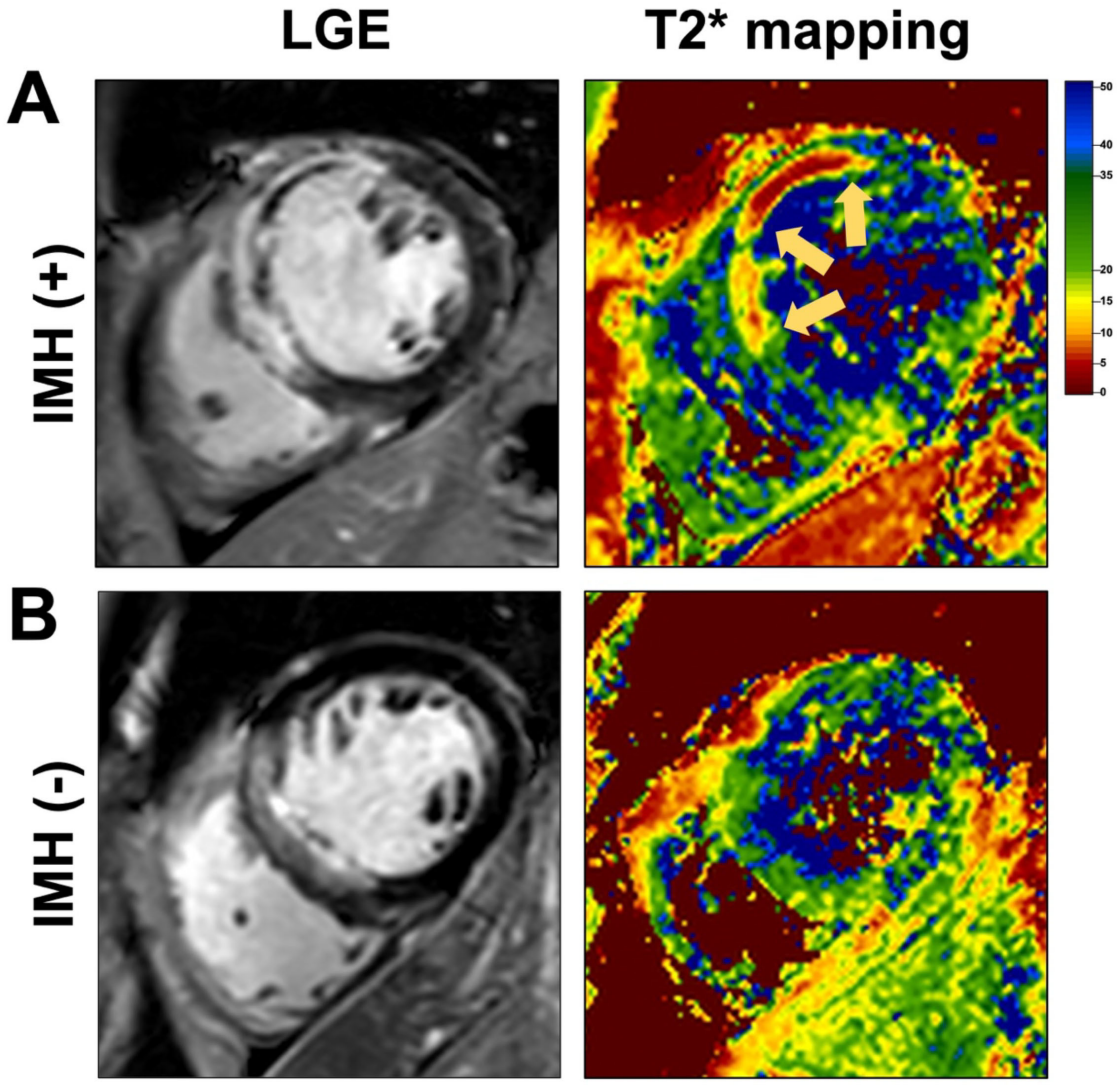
Representative cardiac magnetic resonance (CMR) images of (A) a patient with intramyocardial hemorrhage (IMH) and (B) a patient without IMH. For each case, the left panel shows the late gadolinium enhancement (LGE) image, and the right panel displays the corresponding T2* mapping image for IMH evaluation. IMH is visualized as a hypointense core within the hyperintense infarcted region (indicated by yellow arrows) on T2* mapping cardiovascular magnetic resonance images.
